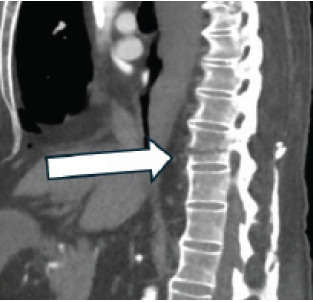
Sagittal computed tomography angiogram of the chest, abdomen, and pelvis (cropped to thoracolumbar region) highlighting an acute chalk-stick fracture (arrow) at thoracic vertebra 11 in a patient with ankylosing spondylitis. This unstable fracture at the thoracolumbar junction was associated with interspinous vascular injury and a large, right-sided hemothorax, underscoring the risk of catastrophic vascular complications in this population.
